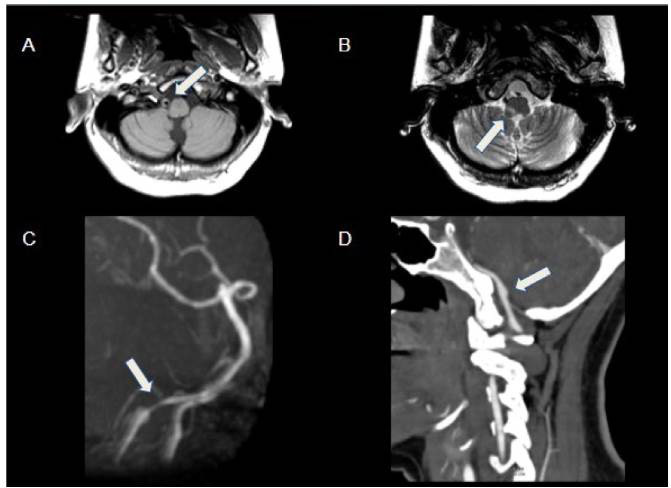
Arterial dissection in 16-year-old female with acute onset of Wallenberg syndrome. Axial T1WI (A) shows high-signal eccentric thickening in the right vertebral artery, compatible with mural hematoma (arrow). T2WI (B) depicts infarcted area in the right dorsolateral portion of the medulla oblongata (arrow). MRA (C) highlights tapering of the V4 segment of the right vertebral artery (arrow), suggestive of dissection. Follow-up CTA (D) shows right V4 dissecting a pseudoaneurysm (arrow).
